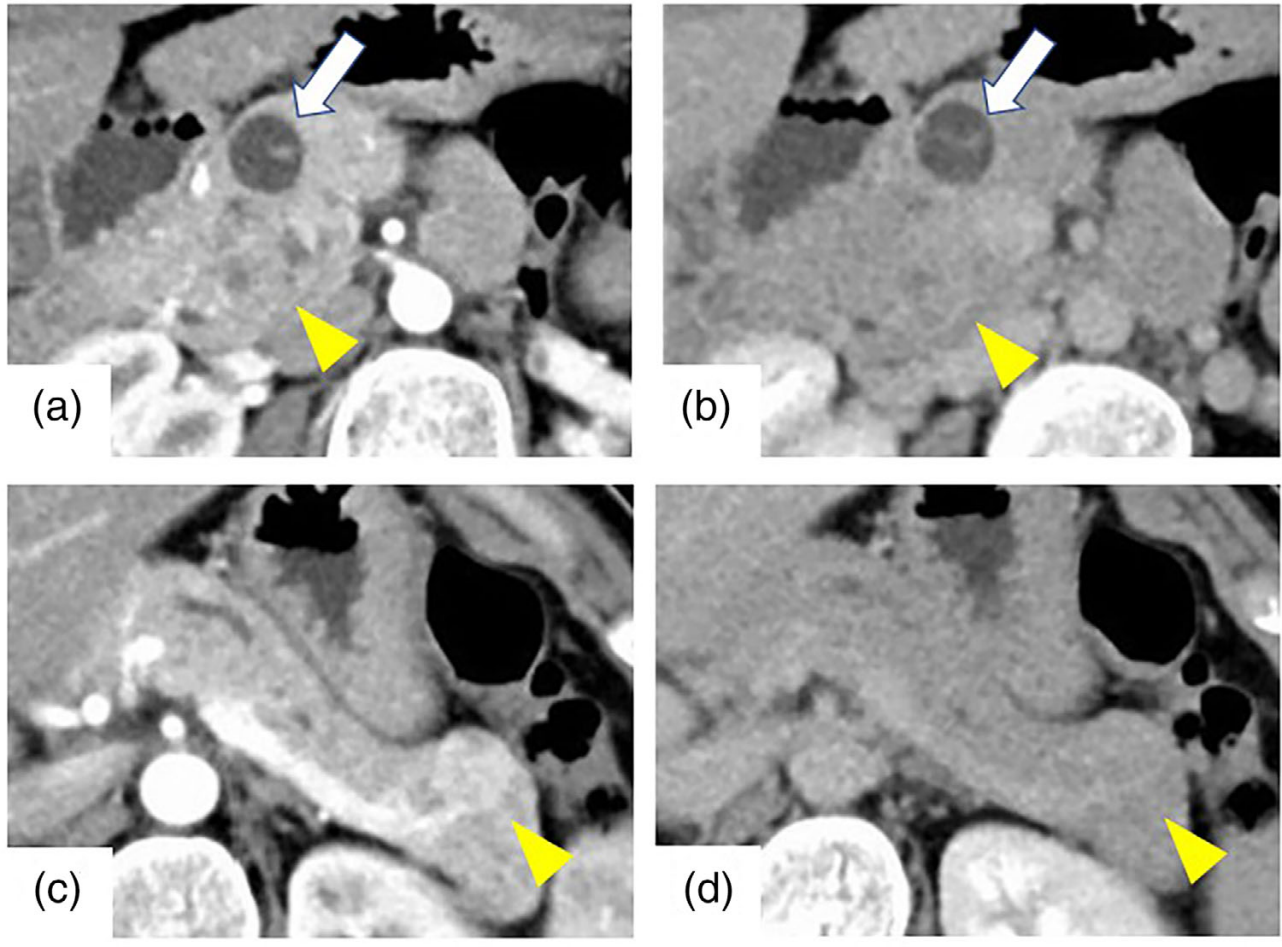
Contrast‐enhanced computed tomography scan. (a) Arterial phase, (b) equilibrium phase: There was a 26‐mm, poorly demarcated, hypodense nodule that exhibited a faint contrast effect in the pancreatic head (yellow arrowhead). There was also a 15‐mm cystic lesion in the pancreatic body (arrow). (c) Arterial phase, (d) equilibrium phase: There was a 17‐mm, well‐demarcated, solid nodule that exhibited a contrast effect during the vascular phase in the pancreatic tail (yellow arrowhead).
